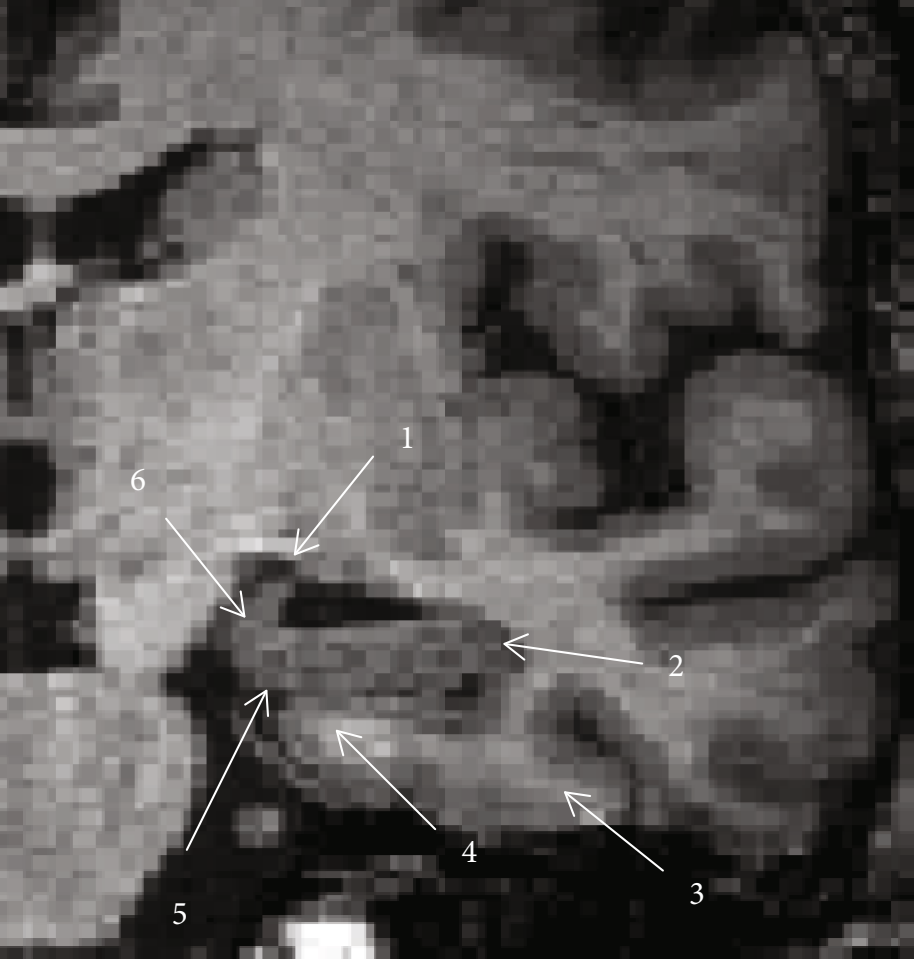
A detailed view of left mediotemporal structures on the optimal slice of brain MRI. Anatomical structures are labeled as follows: 1: hippocampal fimbria; 2: alveus; 3: parahippocampal gyrus; 4: subiculum; 5: hippocampal fissure; 6: uncus of the parahippocampal gyrus.
